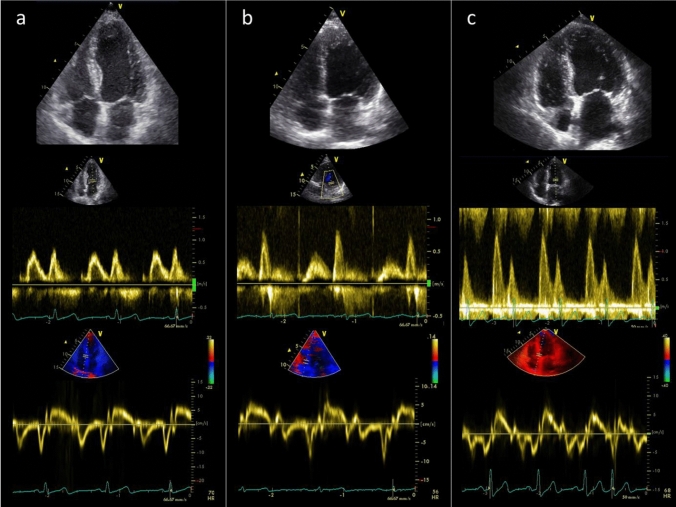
Echocardiographic assessment of cardiac remodelling and diastolic dysfunction in three exemplary female ESKD patients with preserved ejection fraction. Upper, middle, and lower panels respectively show apical 4-chamber view (upper panel), pulsed-wave Doppler evaluated diastolic filling pattern (middle panel), and tissue Doppler early diastolic mitral annular velocity (e´, lower panel). a Apparently healthy heart, LVMi = 59 g/m^2^, LVEF = 72%, LAVi = 33 ml/m^2^, E/e’ = 8.9 b Beginning LV hypertrophy and mild diastolic dysfunction, LVMi = 103 g/m^2^, LVEF = 61%, LAVi = 53 ml/m^2^, E/e’ = 12.6 c Severely enlarged LV and severely impaired diastolic function, LVMi = 147 g/m^2^, LVEF = 67%, LAVi = 56 ml/m^2^, E/e’ = 33.0
